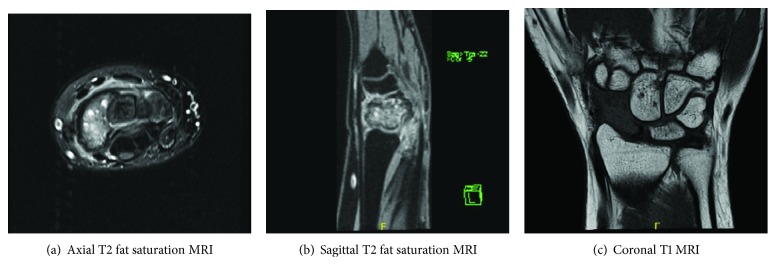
Axial T2 fat saturation (a), sagittal T2 fat saturation (b), and coronal T1 (c) MRI sequences of the right wrist, demonstrating a marrow-replacing bone lesion within the scaphoid.
